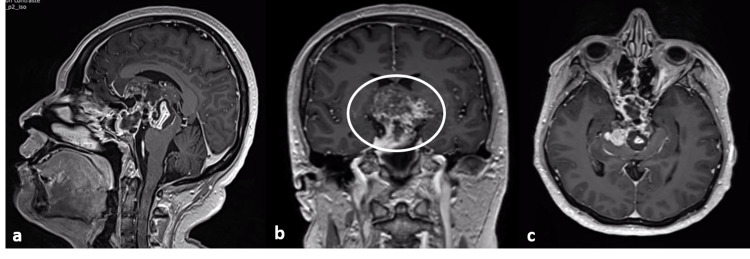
Imaging findings of case two. MRI reconstruction in T1 with gadolinium shows a diffuse heterogeneous sellar mass (a) with multiple densities and walls that give it a cyst-like appearance (oval in b). It pushes the adjacent structures and has a hyperintense capsule-like structure surrounding it (c).
